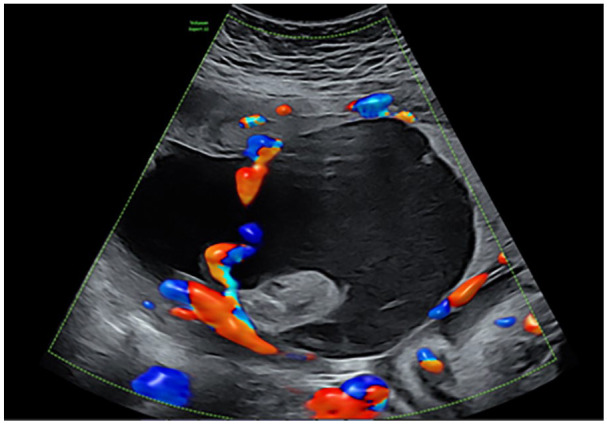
Large cystic placenta structure using Color Doppler, showing no internal vascularity within the structure itself, however abundant blood vessels around the cyst.
